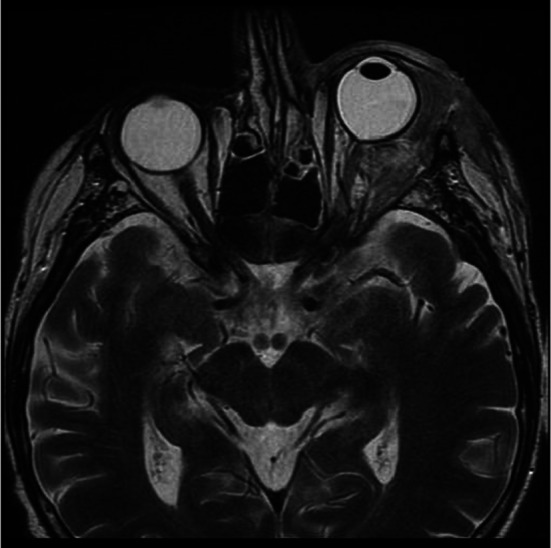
Magnetic resonance imaging orbitals was performed, which showed an image of an abscess of the left orbit, exophthalmos and a forced course of the optic nerve
